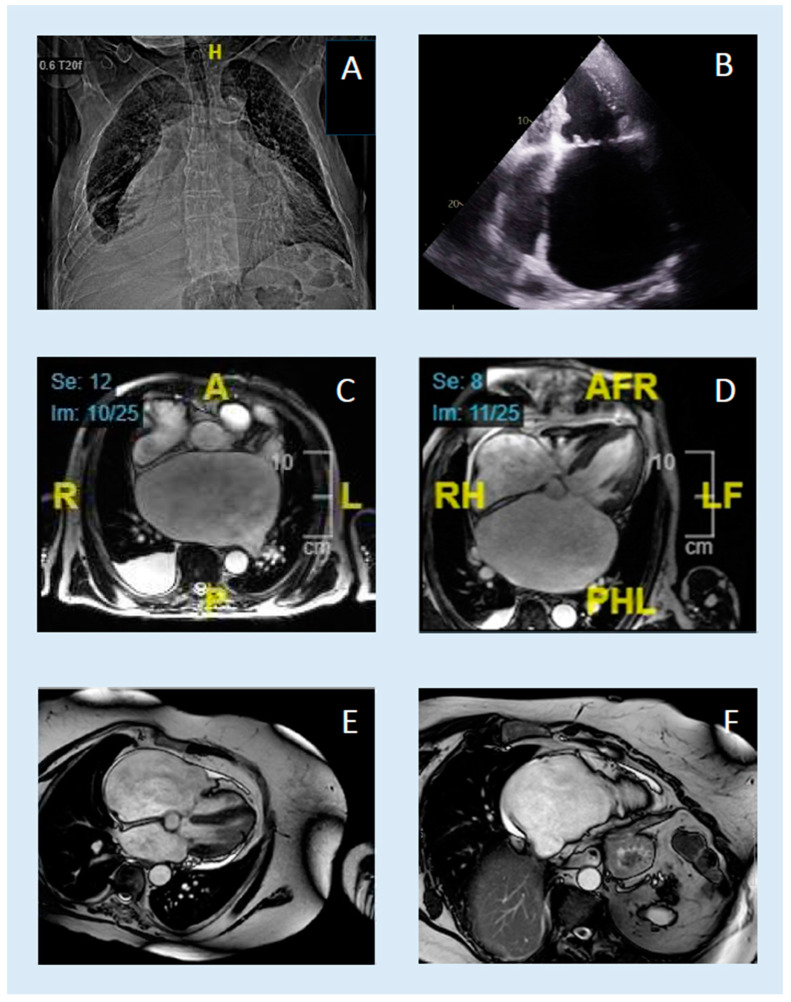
Examples of different imaging methods in “giant atrium“. (A–D): Patient A with *left giant atrium.* (E,F): Patient B with *right giant atrium.* A: Chest X-ray ap; B: Thansthoracic echocardiography apical 4-chamber view in endsystole; C: transversal bSSFP-cine CMR in endsystole; D: 4-chamber bSSFP-cine CMR in endsystole; E: 4-chamber bSSFP-cine CMR in endsystole; F: right focussed 2-chamber bSSFP-cine CMR in endsystole. H: head; F: feet; A: anterior; P: posterior; R: right; L: left.
